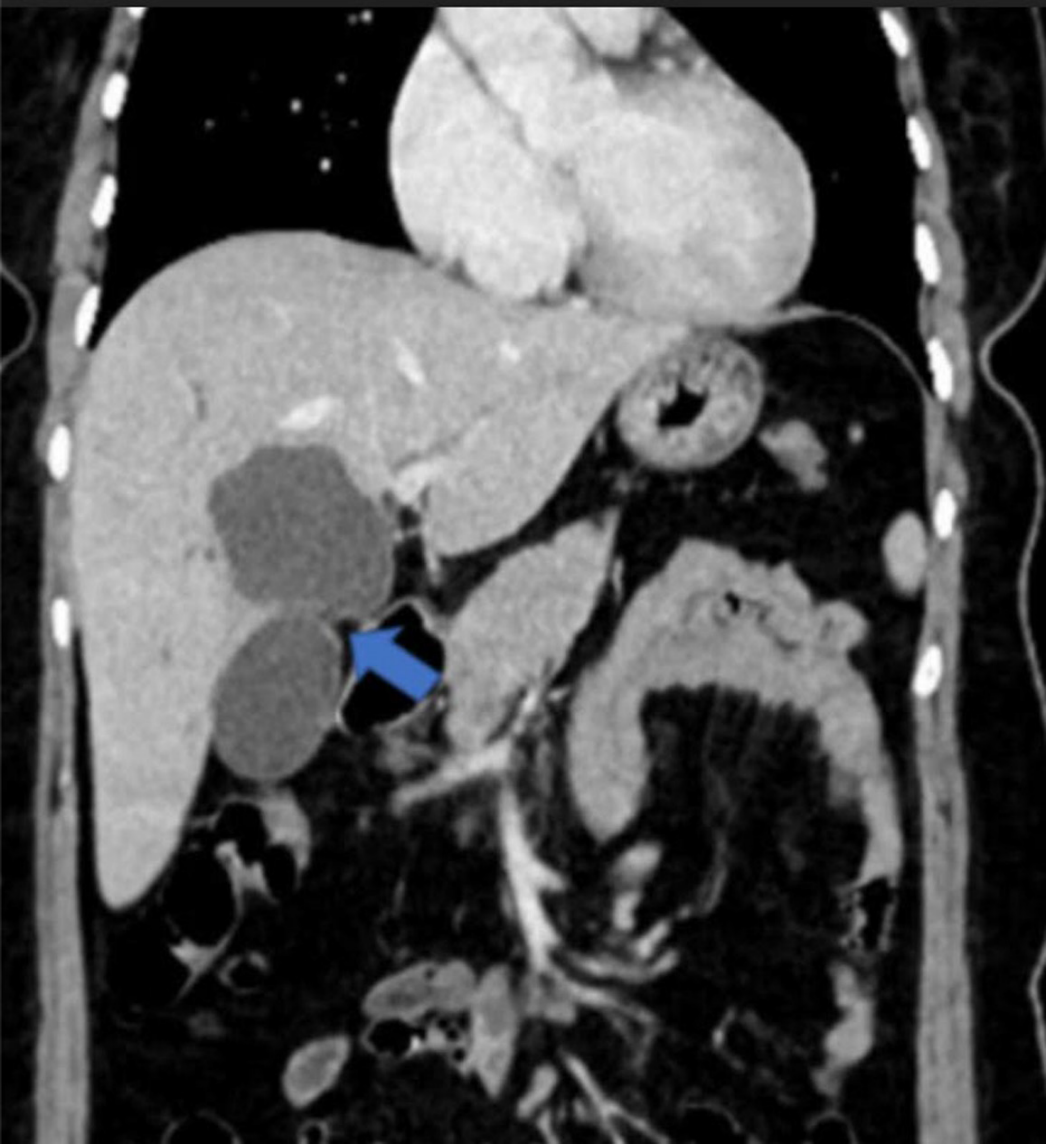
CT coronal section in venous phase shows intrahepatic cystic lesion.
